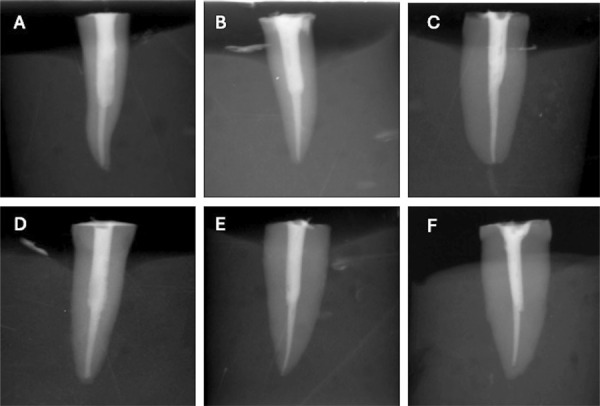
Periapical radiographs of the teeth with root canal filling after removal of the broken instrument. A: GFU, B: GFB, C: GPU, D: UFU, E: UFB, F: UPU.
